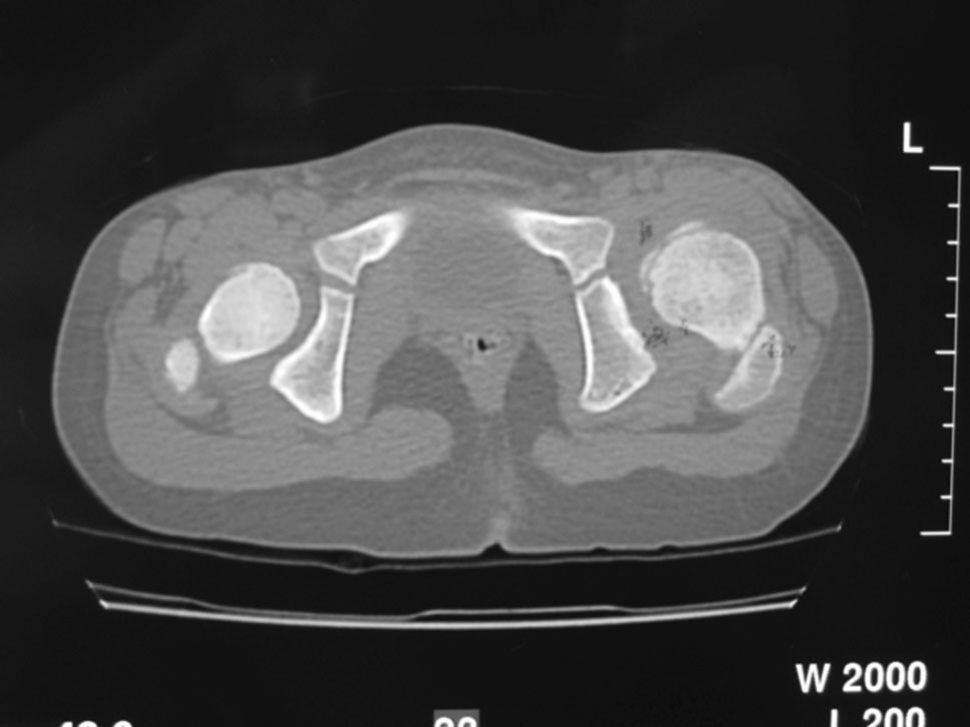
A single-cut computed tomography scan is performed postoperatively to check the adequacy of reduction
